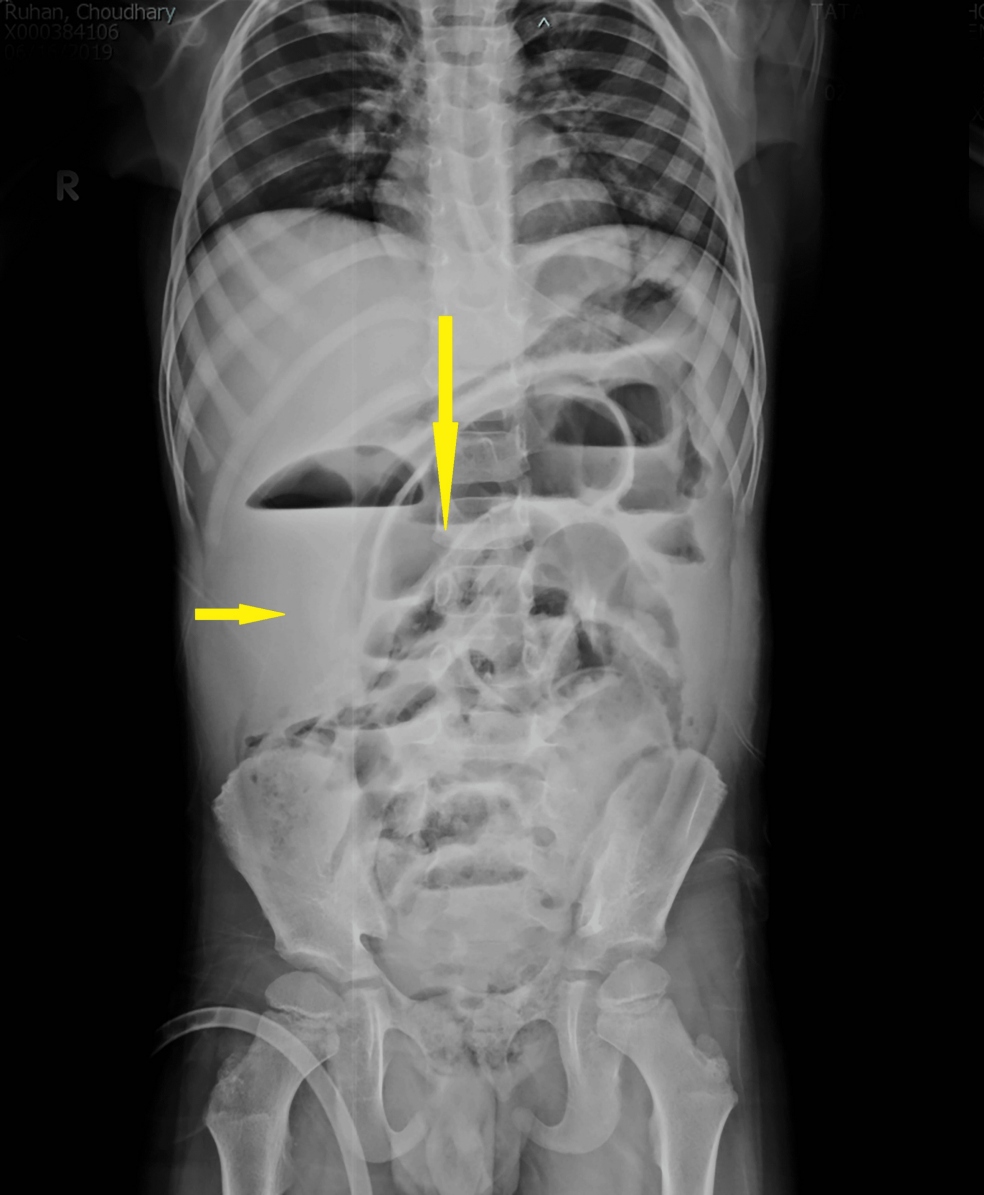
Erect X-ray of the abdomen. Collection in the right iliac fossa (horizontal arrow) and periumbilical region, along with clumped-up small bowel loops (vertical arrow).
